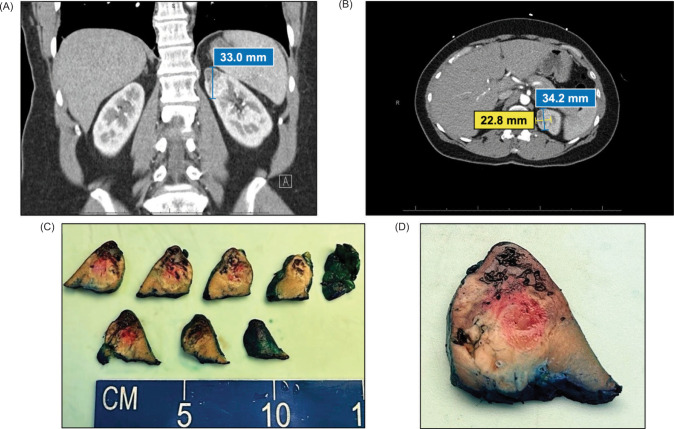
Radiographic and gross images. (A) Coronal CT of abdomen with left kidney mass measuring up to 33.0 mm; (B) Axial CT of abdomen with left kidney mass measuring 34.2 × 22.8 mm; (C) Serial sectioning of the left superior pole kidney mass; (D) Close-up image of the poorly defined mass.
